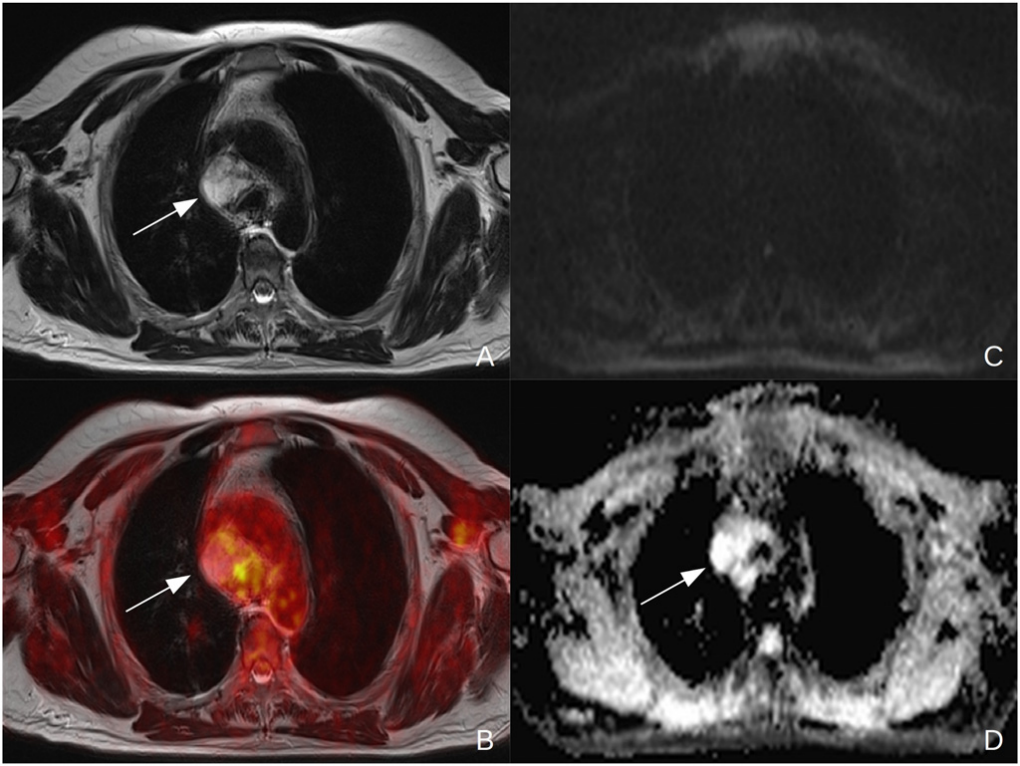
55y old male patient suffering from NSCLC (tumor stage IV, adenocarcinoma, G2) Central necrotic lymph node in the mediastinum (see arrow) with a high T2 signal in MRI (A) and a low FDG-uptake in the fused PET/MR images (B). In DWI, no diffusion restriction can be observed (C) and a T2 shine through effect can be seen on the corresponding ADC-map (D).
